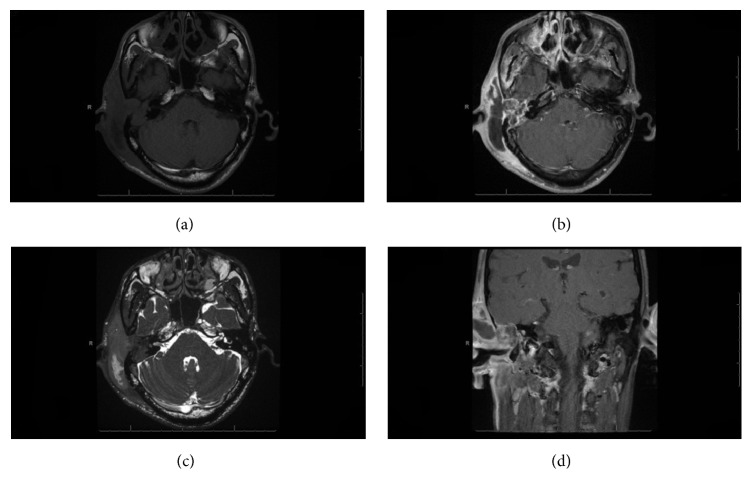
MRI of the brain, IAC, and mastoid with IV contrast. Axial and coronal views show (a) MRI T1 axial view before contrast, (b, c) MRI T1 axial view after contrast, and (d) MRI T1 coronal view after contrast. Right, large, loculated, peripheral enhancing collection is observed in the subcutaneous tissue of the auricular region, posterior and anterosuperior to the external auditory canal and extending to the mastoid air cells and middle ear cavity. The cochlea and semicircular canals are not visualized; only part of the vestibule is observed, and a focal area of dural enhancement is observed in the right temporal lobe.
